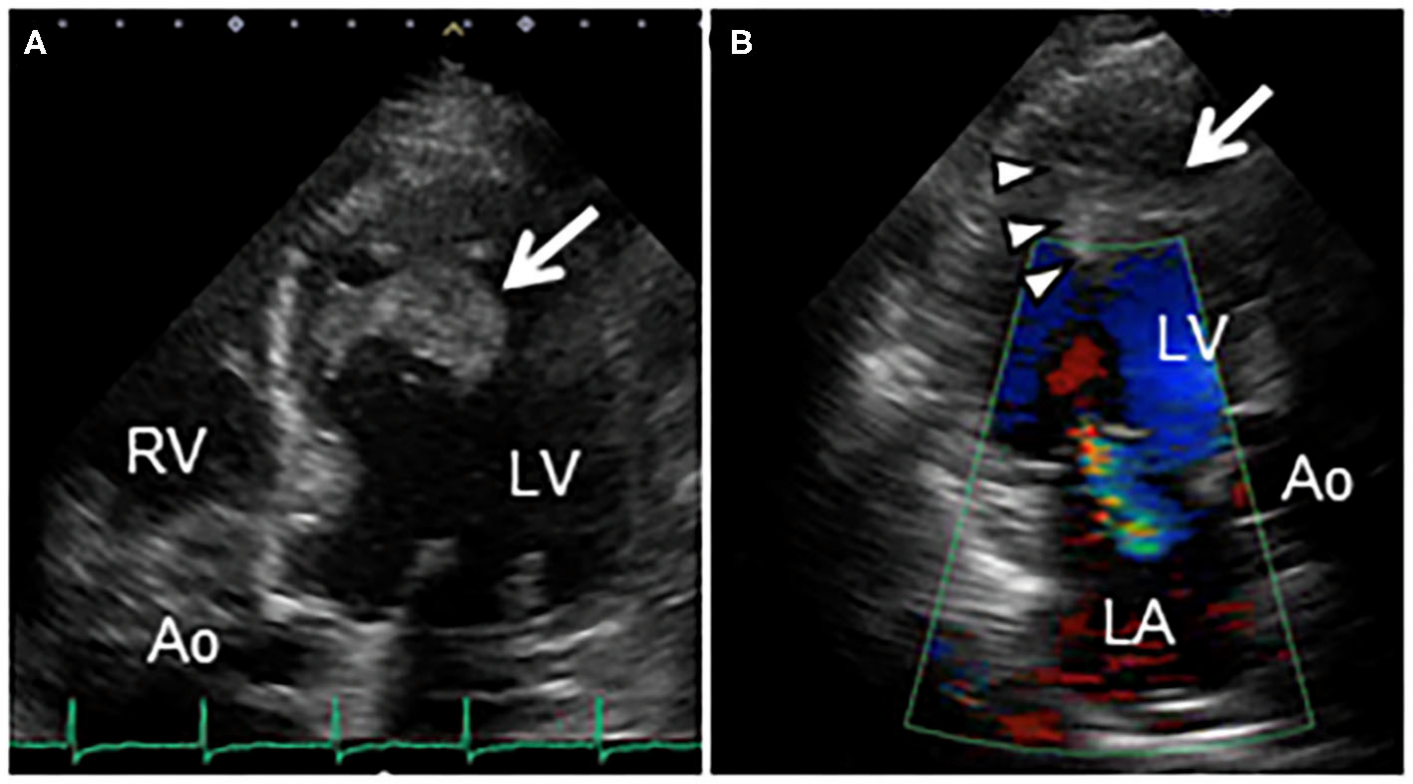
(A) Transthoracic echocardiography in apical 5-chamber view reveals an echo-dense mass (30 × 39 mm, arrow) attached to the mid-anteroseptal wall of the left ventricle, extending to the anterolateral papillary muscle. (B) Color Doppler echocardiography in apical 3-chamber view reveals a cardiac mass (arrow), and entrapment of the anterolateral papillary muscle (arrowheads), causing moderate mitral regurgitation. Ao, Aorta; LA, Left atrium; LV, Left ventricle; RV, Right ventricle.
